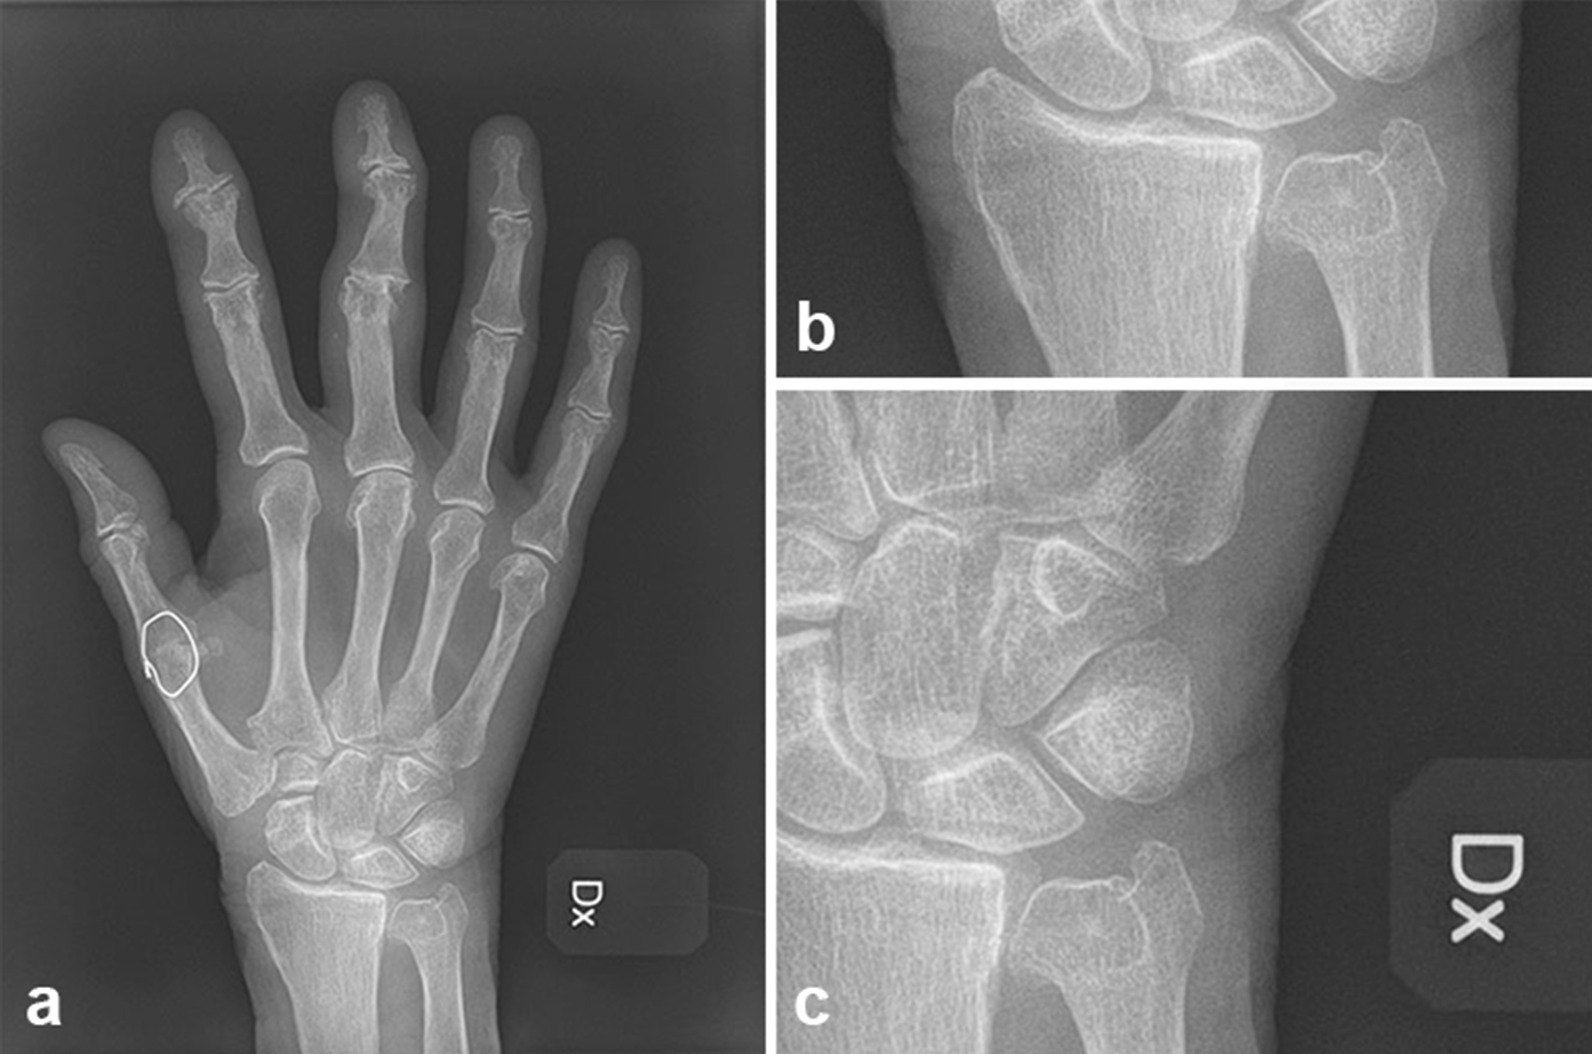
Radiograph of wrist 18 years after removal of the trapezium, before blinding (a), and after blinding (b, c)
